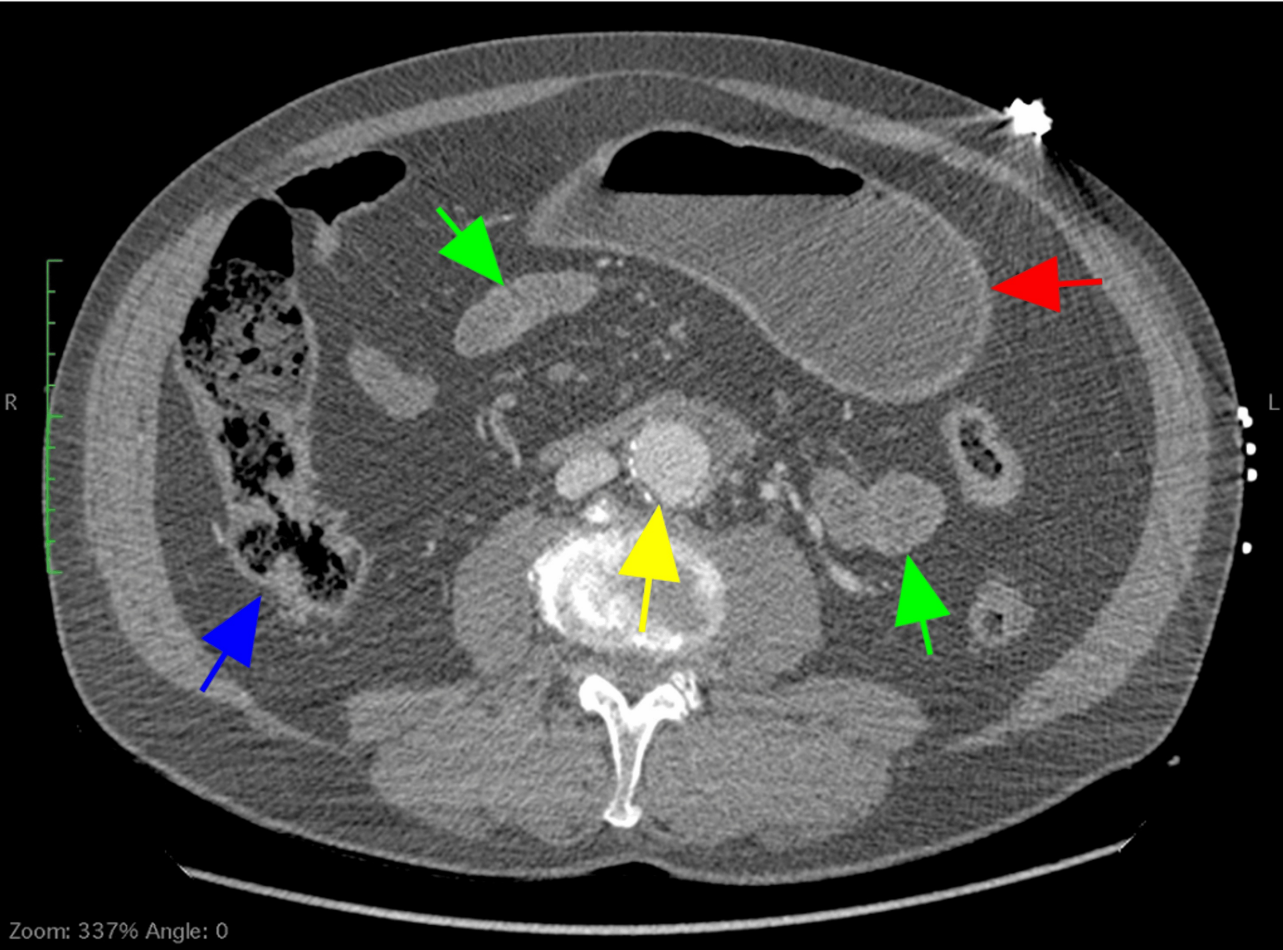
Contrast-enhanced computed tomography scan showing only a mildly dilated stomach (red arrow), and normal appearance of the small (green arrow) and large bowel (blue arrow). An infrarenal abdominal aortic aneurysm is also noted (yellow arrow).
